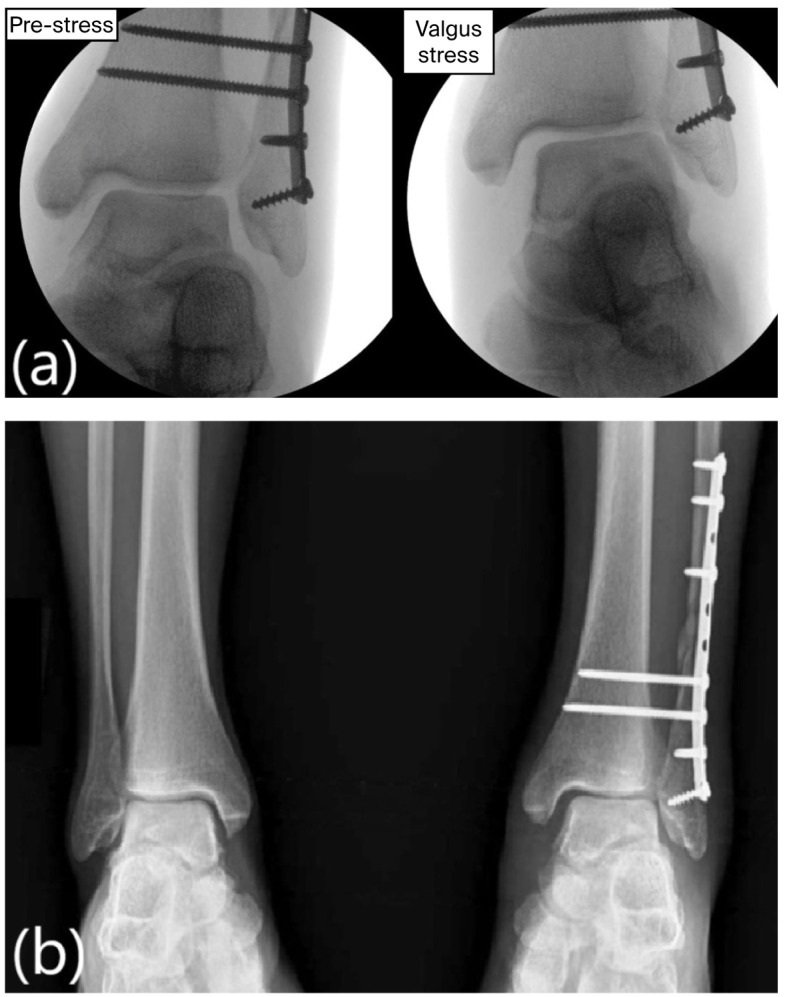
Stress fluoroscopy (a) in the office at week 8 postop showing persistent medial instability. Bilateral standing AP radiograph (b) showing subtle widening of medial clear space and fibular shortening.
